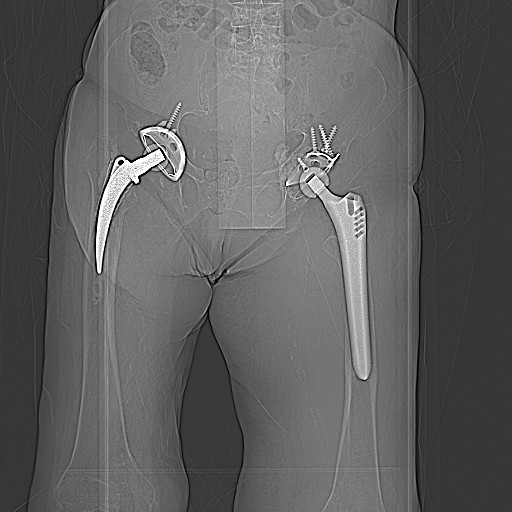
CT-scout-view showing the nondisplaced anterior pelvic ring fracture on the left side.
